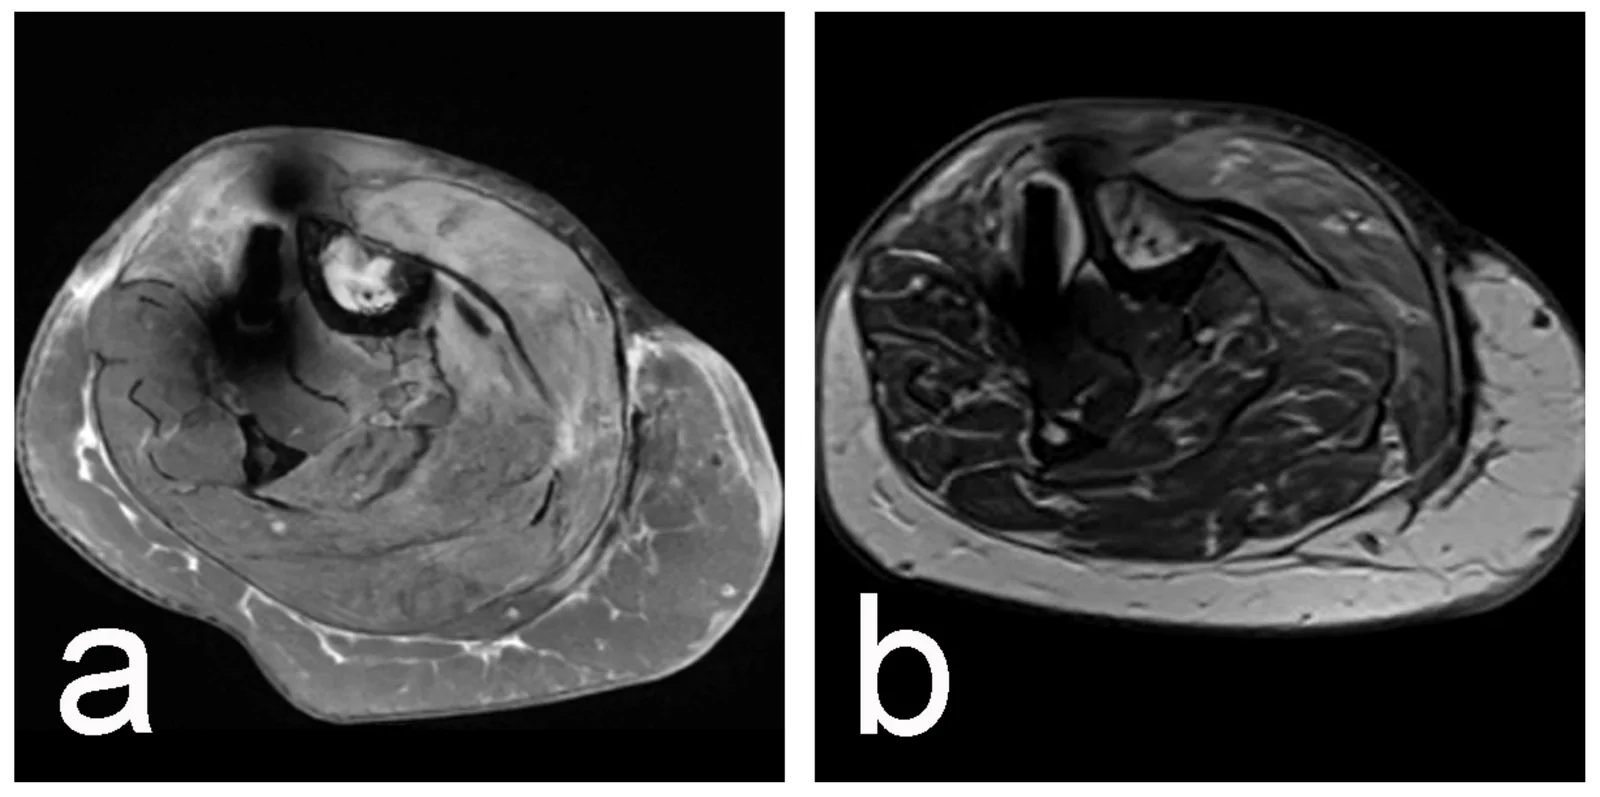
Postoperative contrast-enhanced MRI (a,b) showing the anteromedial tibial cortical defect, plate-and-screw stabilization, and reconstructed soft tissues.
